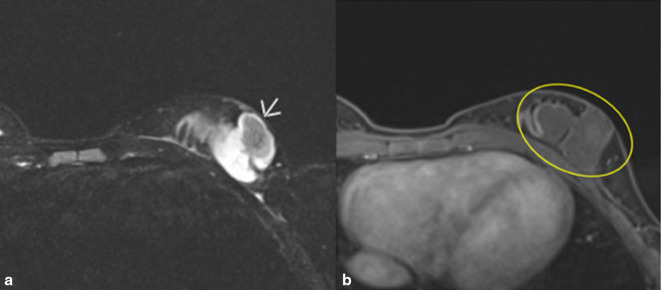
A and B. MRI images of left breast with contrast. (A). *T_2_*-weighted axial fat-suppressed sequence. Arrow shows a lobulated cystic multioculated mass in the left lower outer breast measuring 4.9× 2.7 × 5.6 cm superior to the patient’s scar consistent with lymphatic malformation. (B). T1 axial postcontrast and fat-suppressed sequence. No significant internal enhancement. Minimal rim enhancement. Mass goes to skin and close proximity to chest wall without invasion.
